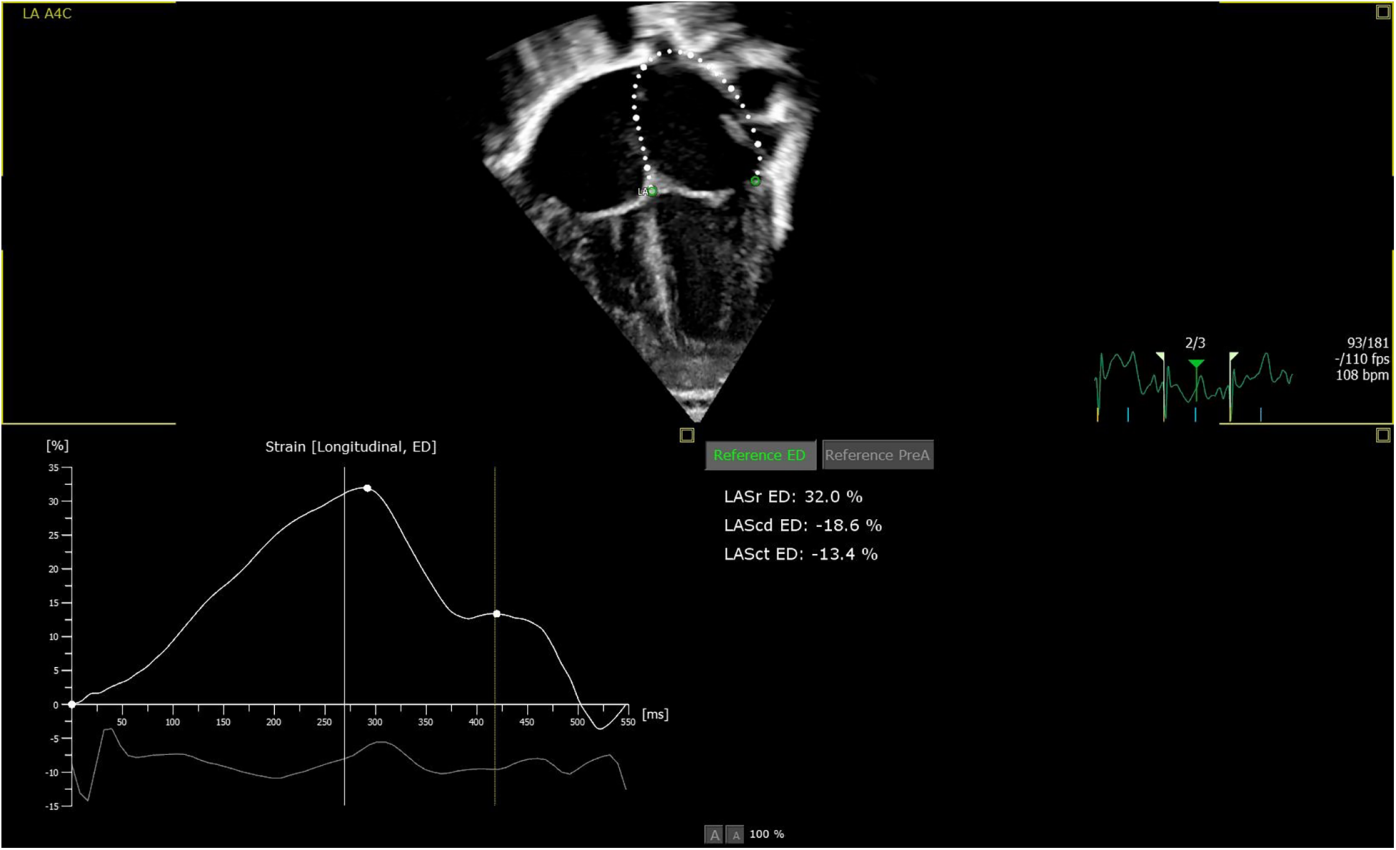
Picture of apical four chamber view: software automatically measuring LASr, defining the endocardial border as region of interest, excluding the pulmonary veins and/or LA appendage orifice.
